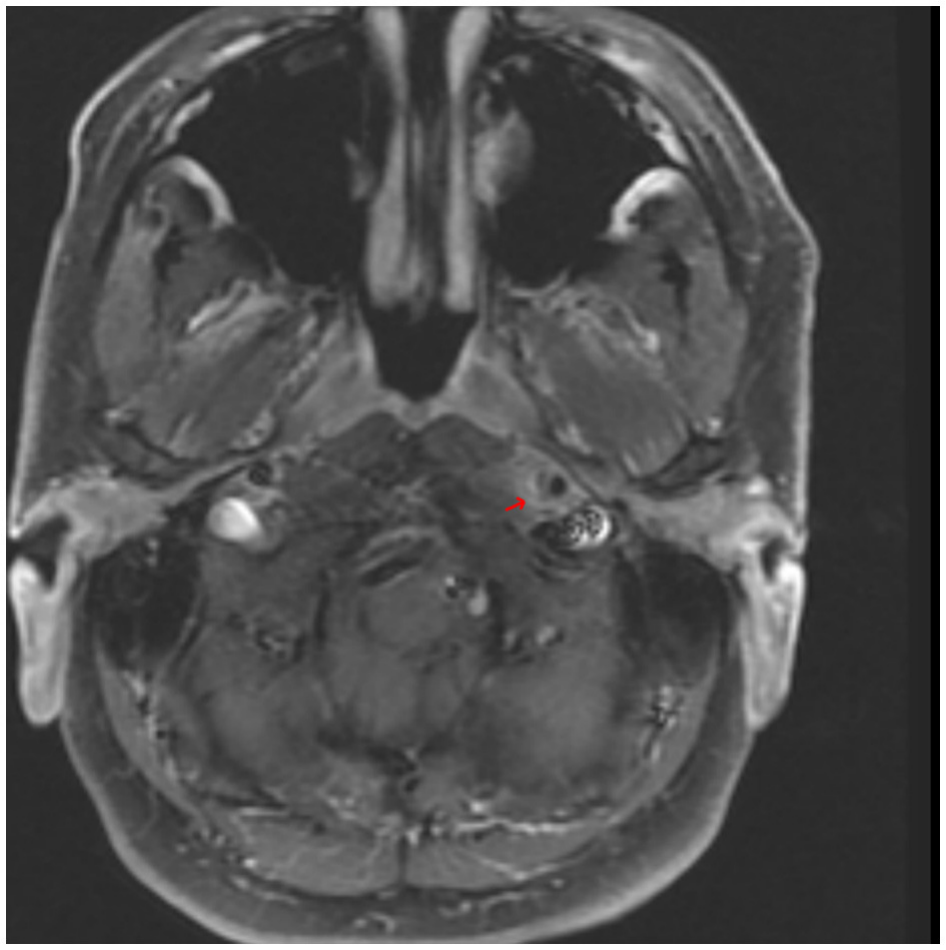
An axial T1-weighted MR angiography post-gadolinium image demonstrating hyperintensity surrounding the lower left ICA near the bifurcation and corresponding to the internal carotid artery wall; arrow indicates area of suspected perivascular enhancement in the region of the dissection in the distal cervical segment.
